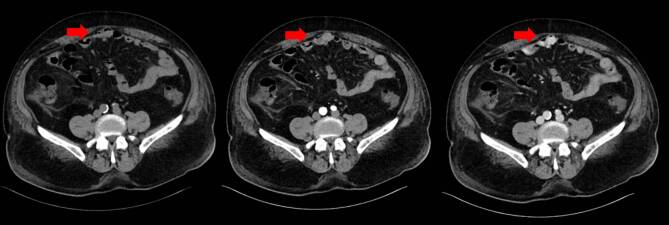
Abdominal computed tomography in transversal plane, showing the unenhanced (left), the arterial contrast enhanced (middle) and the venous sequence (right) of the examination. The venous sequence shows “pooling” as a sign of contrast extravasation into the small intestine, representing active bleeding into the jejunal lumen (*arrows*)
